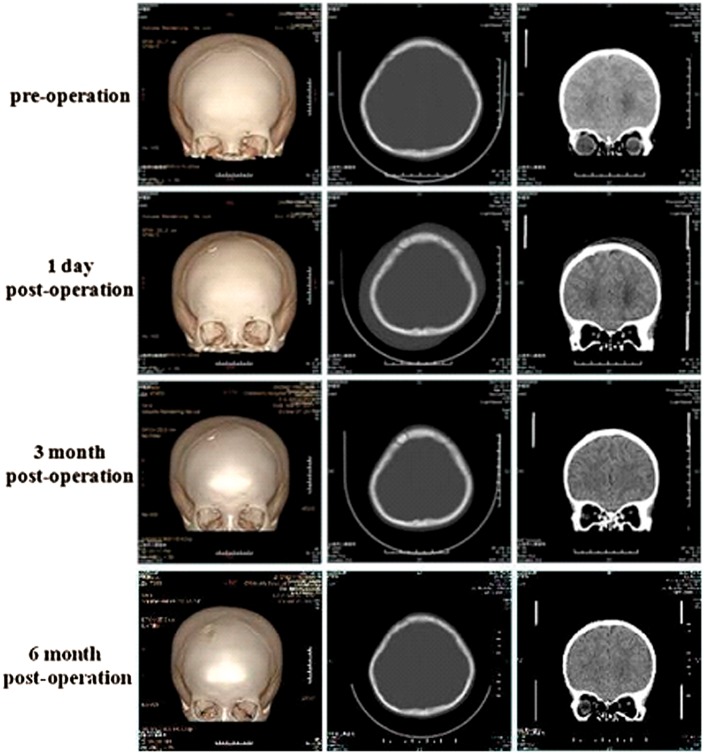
The skull CT scan before and after operation
